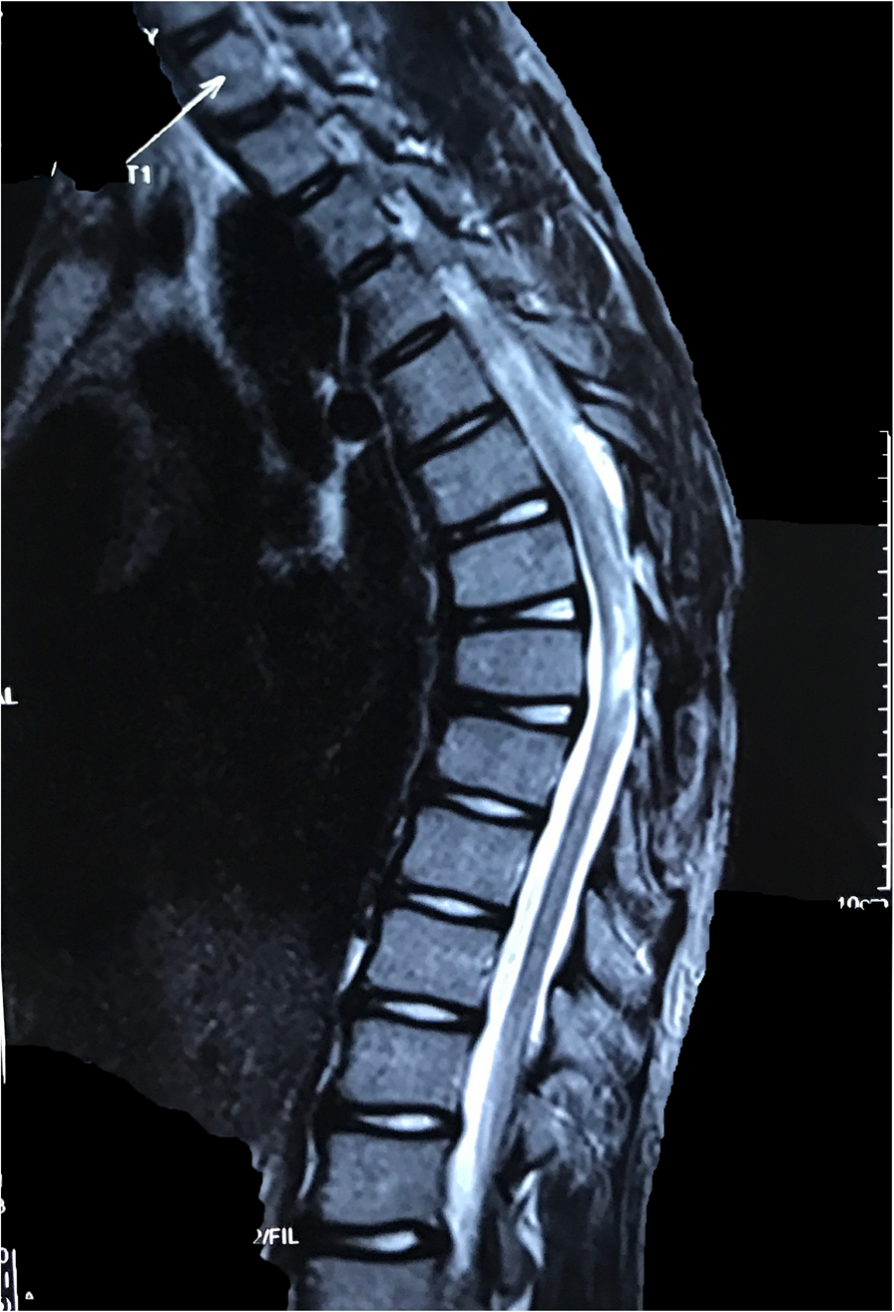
Postoperative MRI did not reveal the presence of a residual tumor and a progressive spinal kyphosis was observed 3 years after surgery
